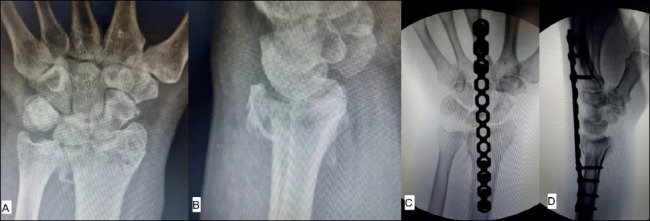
Preoperative radiographic images (A and B). Intraoperative fluoroscopic images after reduction and fixation (C and D).
